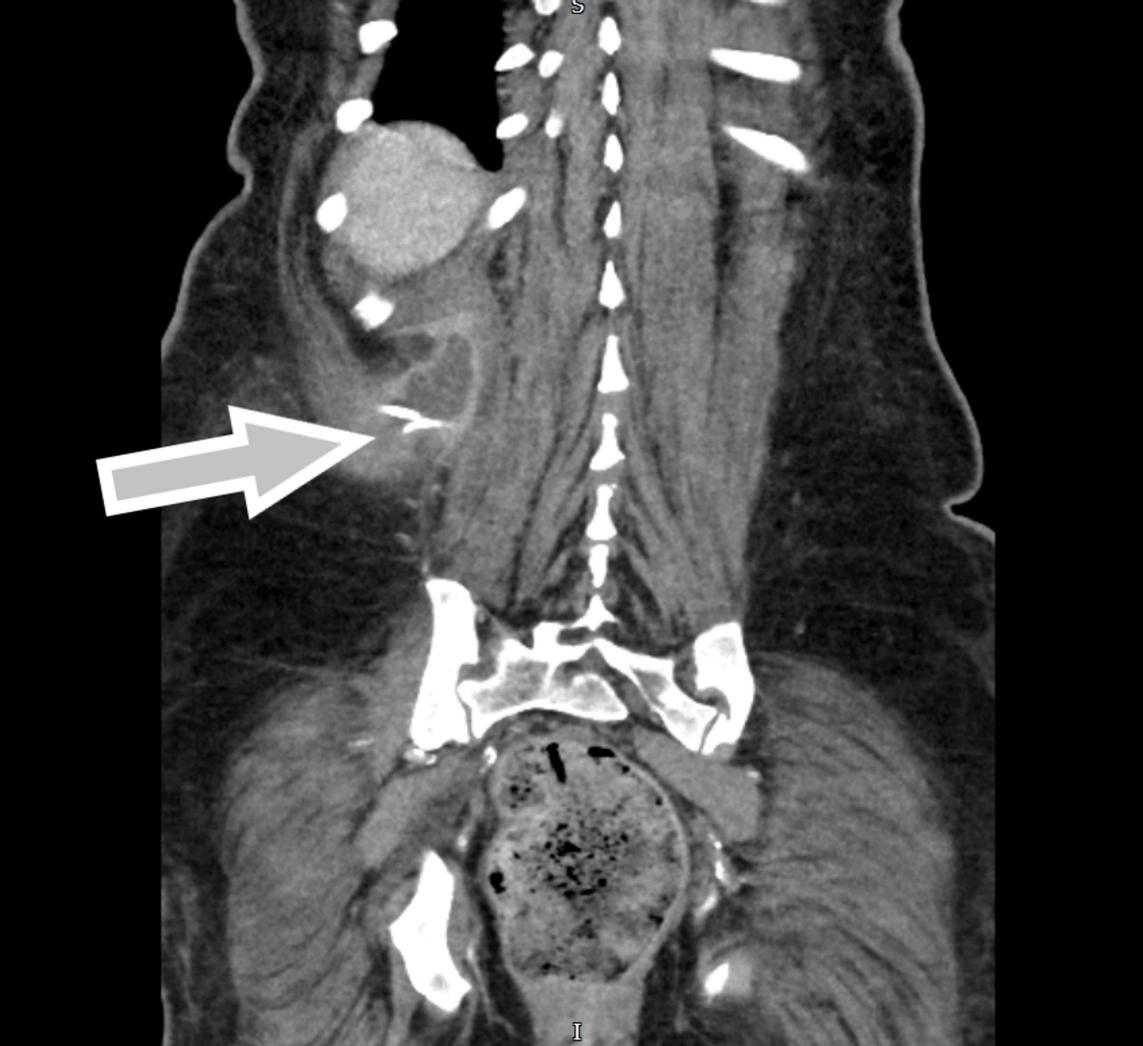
CT abdomen pelvis with contrast following drain insertion shows pigtail catheter within the right perinephric collection, extending into the paravertebral muscles CT: computed tomography
